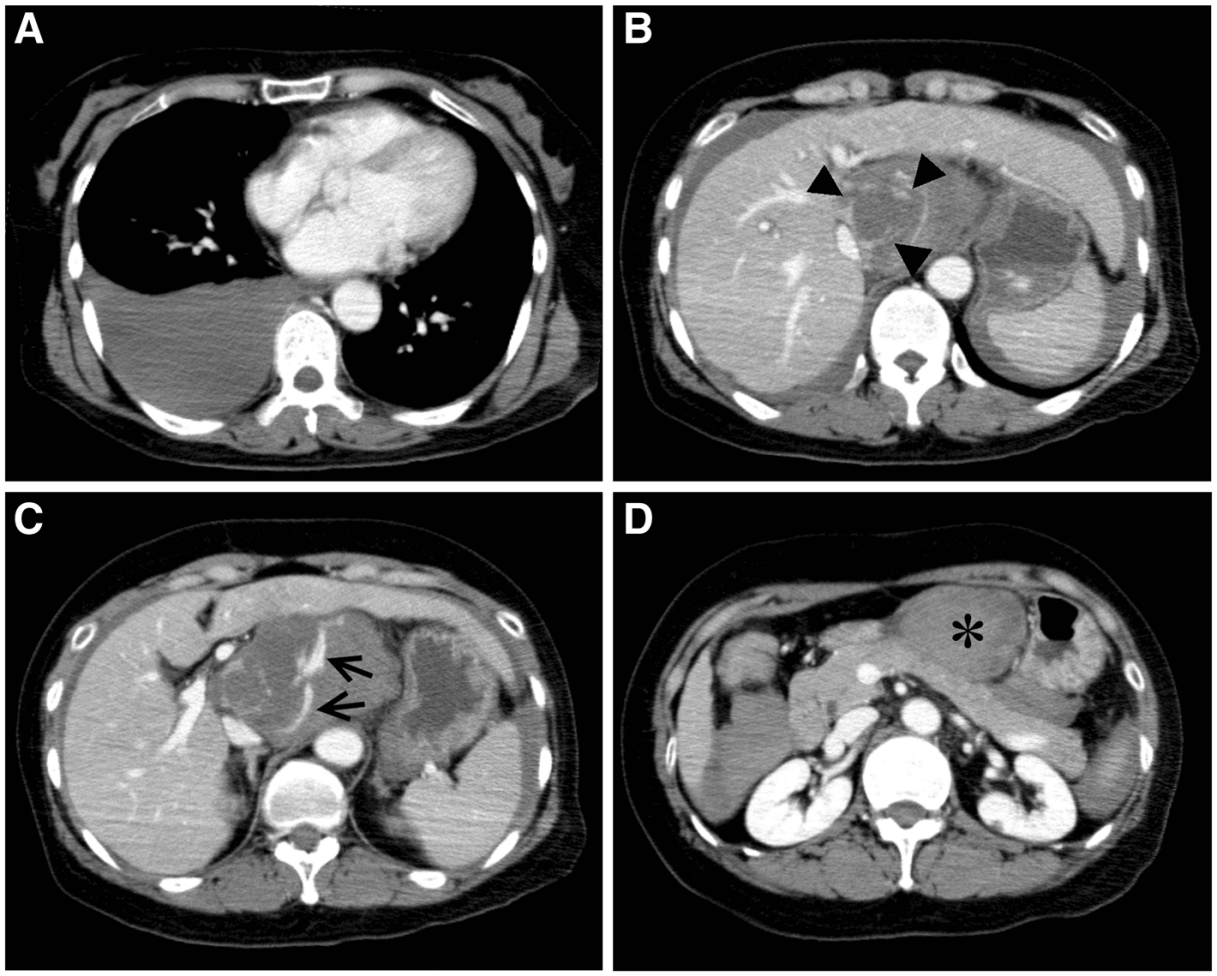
Chest and abdominal contrast-enhanced computed tomography. A) Right hemothorax. B) A ruptured tumor (*arrowheads*) and hemoperitoneum. C) Extravasation of the contrast medium (*arrows*). D) Hematoma inside the omental bursa (*).
